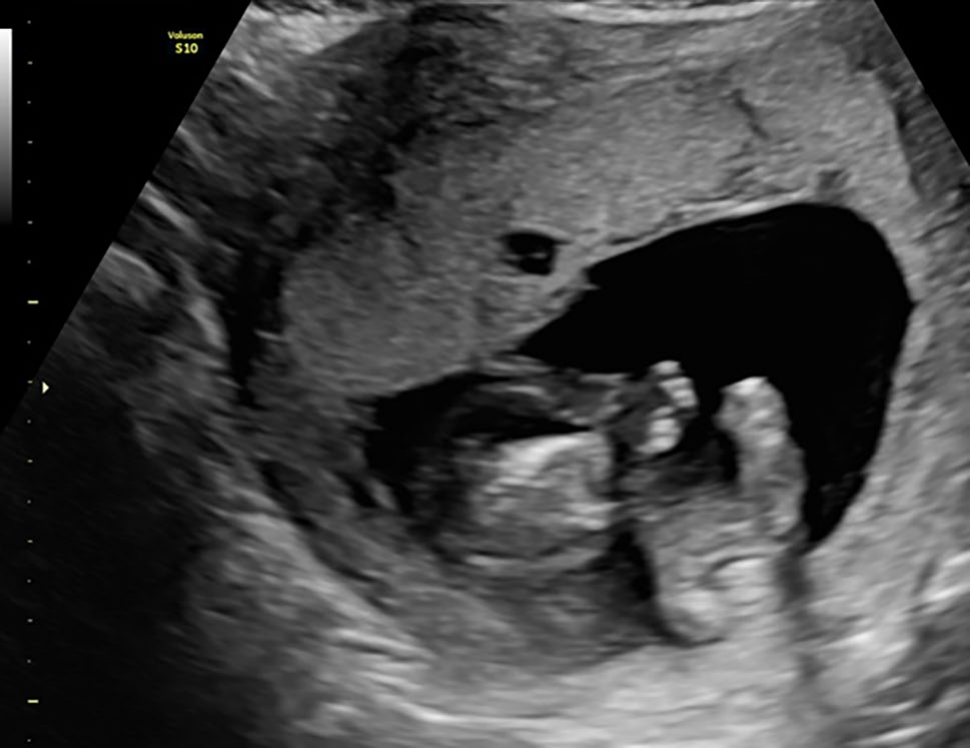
Perinatology team assessment: viable fetus (left): the nasal bone, the stomach, and bladder were observed; The lower and upper extremities were observed as active. No tricuspid valve regurgitation and abnormal waveforms in fetal ductus venous flow assessment were monitored; a single umbilical artery was detected in the fetal umbilical cord. Co-twin (right) shows that the fetus has acrania and acardia, and the upper extremities were not visualized. The spine and lower extremities are observed, but intraabdominal organs could not be detected. Circulation was observed in the acardiac twin, and a Uterine arterial Doppler ultrasound assessment found both the left and right uterine Doppler early diastolic notch
